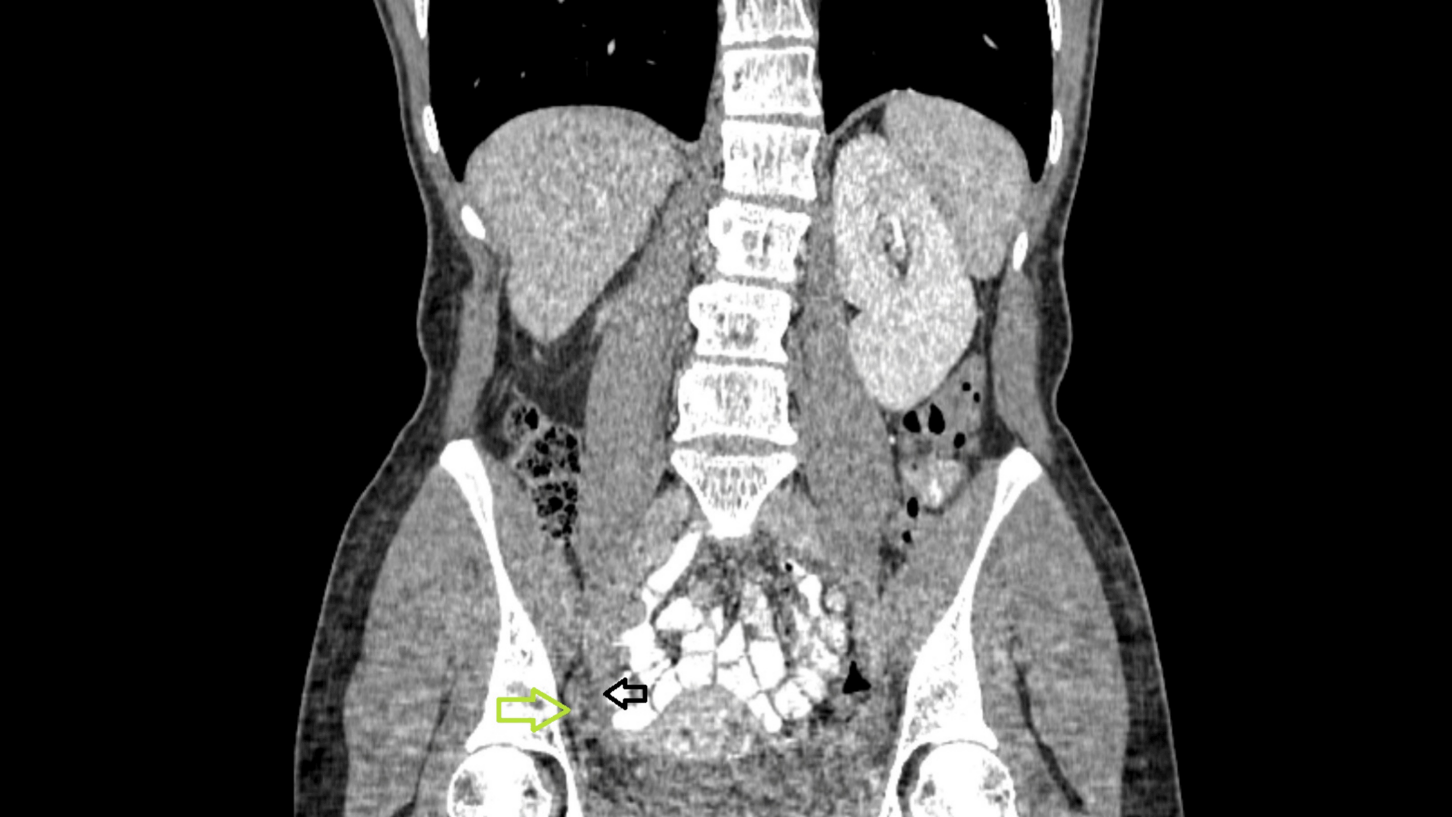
Computed tomography imaging with intravenous and oral contrast in coronal view The close association between the appendix (green arrow) and the broad ligament (black arrow)
